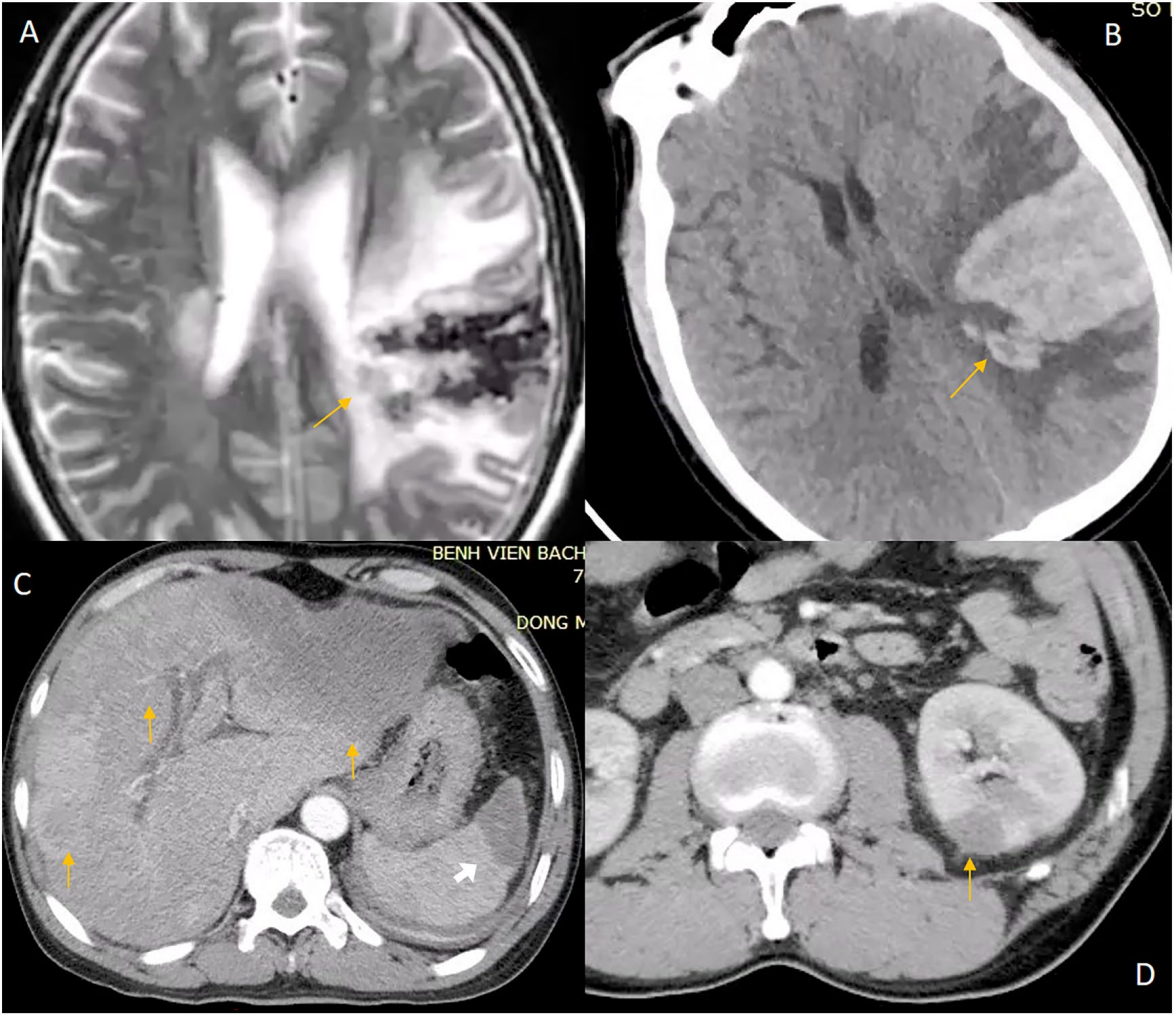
Extracardiac manifestations. (A) T2 weighted magnetic resonance imaging showing cerebral infarction with hemorrhagic transformation. (B) The same lesion on non‐contrast CT. Abdominal multi‐slice computed tomography (arterial phase) image showing: (C) Hypointense masses in left and right lobes of the liver (yellow arrows) and spleen 535 (white arrow). (D) Hypointense mass in the left kidney.
